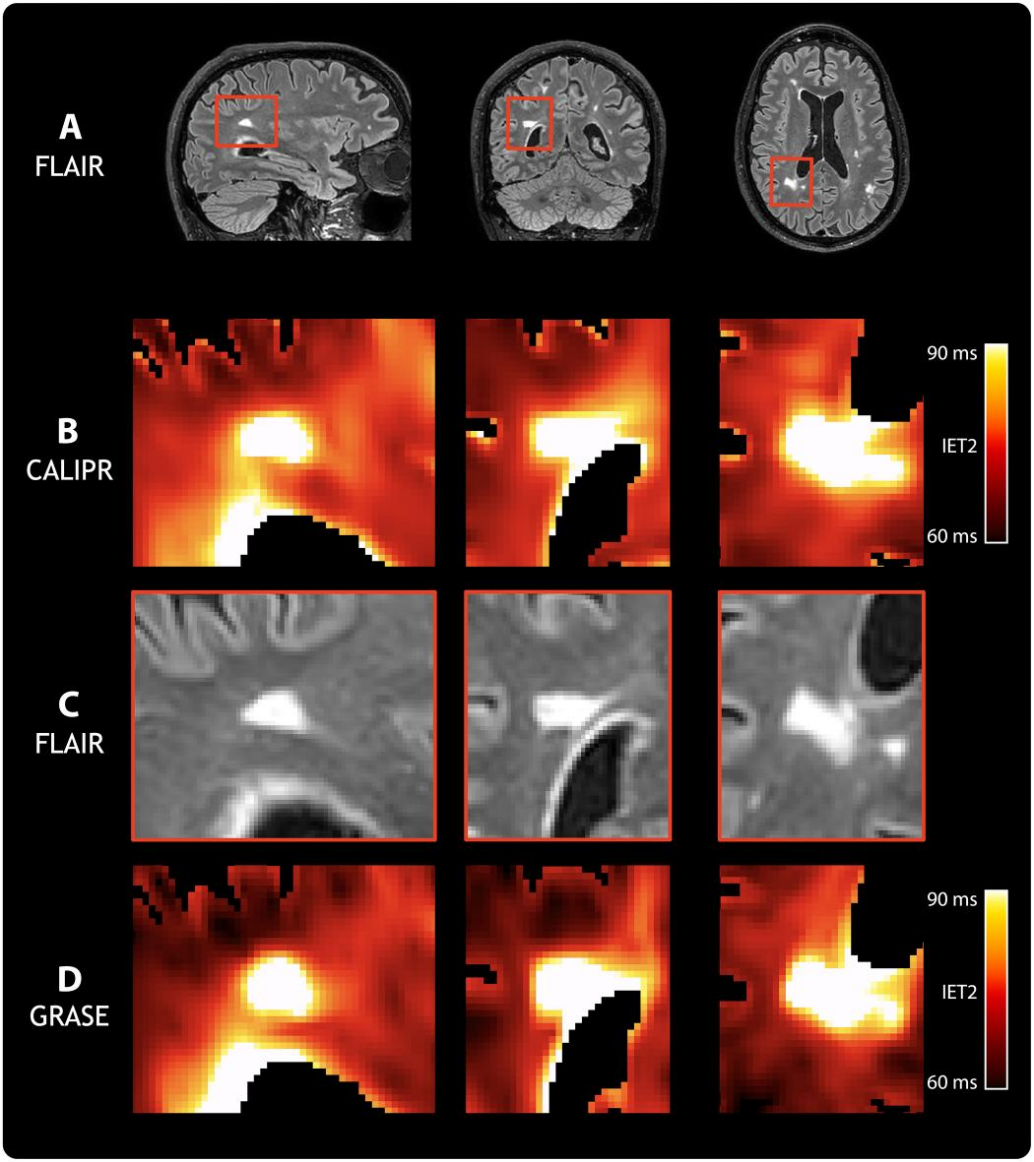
Comparison of geometric mean of IET2 sensitivity to demyelinating disease pathology. From left to right, columns show sagittal, coronal, and axial orientation views, respectively, for a subject living with relapsing-remitting multiple sclerosis (male, age 60 years, expanded disability status scale of 2.0, and disease duration of 12 years). (A) Full slices of a FLAIR anatomical image are shown with red boxes highlighting a region centered around a particularly large FLAIR hyperintense lesion. Close-up views of this region are shown in (B) for IET2 from our proposed CALIPR MWI approach, in (C) for FLAIR, and in (D) for IET2 from a commonly used GRASE MWI approach. All images and maps were aligned in the subject’s T_1_-weighted image space to ensure that the exact same region could be compared.
